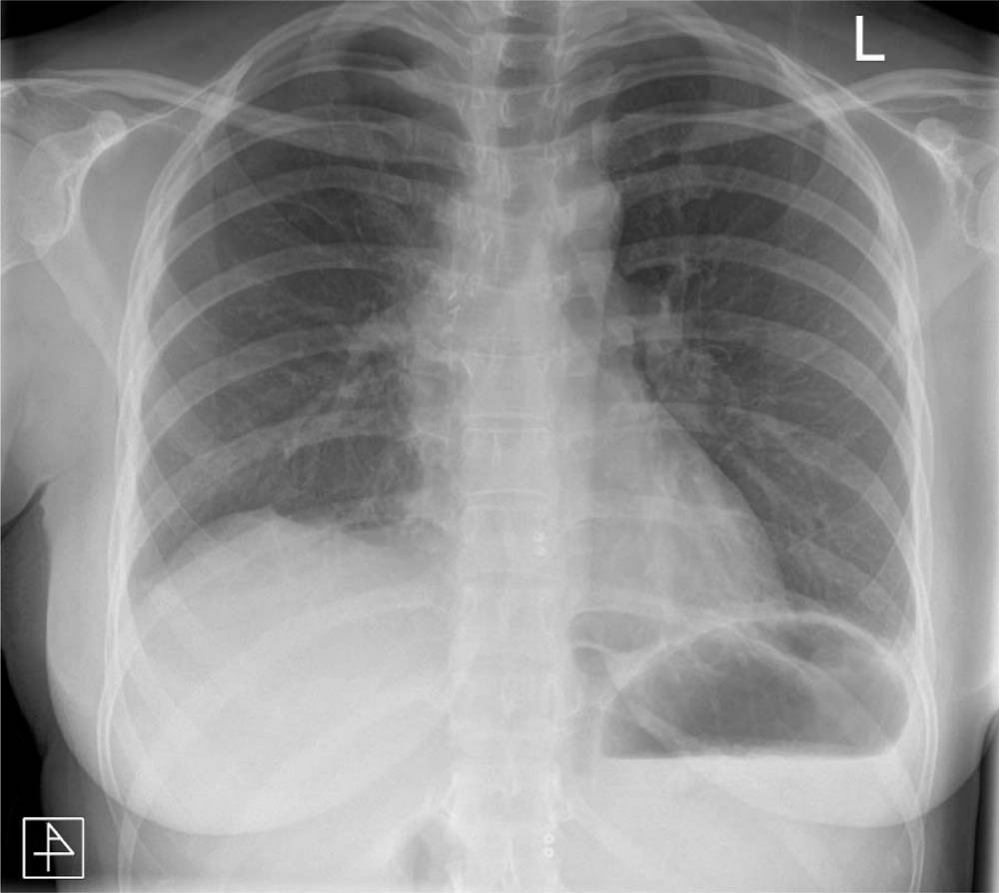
Anteroposterior post-operative chest radiograph.
